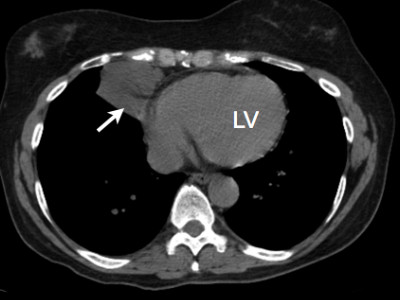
Case 1 -Axial CT image of this patient's thorax demonstrates a lesion in the right anterior cardiophrenic angle. The attenuation of the contents measured 19.6 Hounsfield Units (HU), or near water density.
